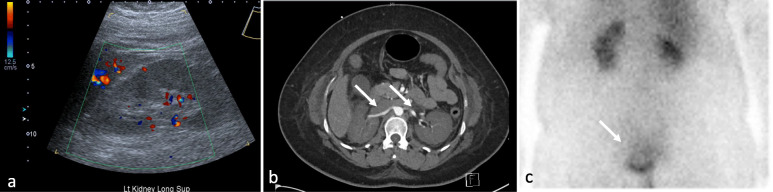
a-c Renal imaging. Left to right. 2a Ultrasound: Bilateral poor parenchymal perfusion, and poor visualisation of renal arteries and veins. 2b CT angiogram: patent renal arteries with bilateral poor parenchymal perfusion. Renal veins appear patent. 2c MAG3 scan: while markedly reduced renal excretion, there is evidence of some small degree of residual function as demonstrated by tracer excretion into the urinary bladder
